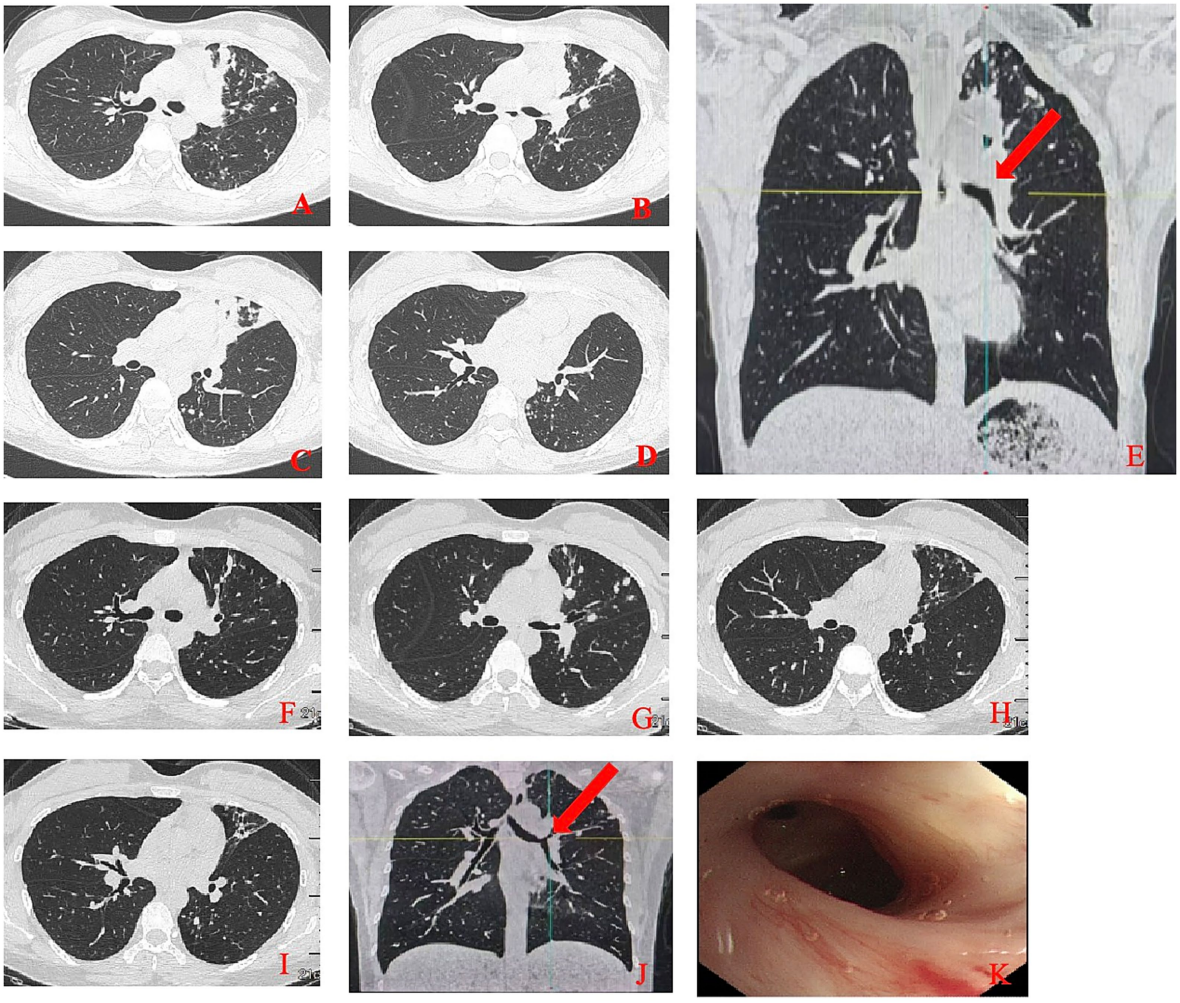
Initial and follow-up chest CT Imaging with corresponding bronchoscopic findings. (A–E) Initial chest CT scan showed complete occlusion (arrow, E) of the left upper lobe bronchus and multiple infectious lesions in the left lung. (E–J) Follow-up chest CT showed significant resolution of the lesion (arrow, J) with a patent left upper lobe bronchus. (K) The left upper lobe bronchus with smooth mucosa and stable scar tissue.
